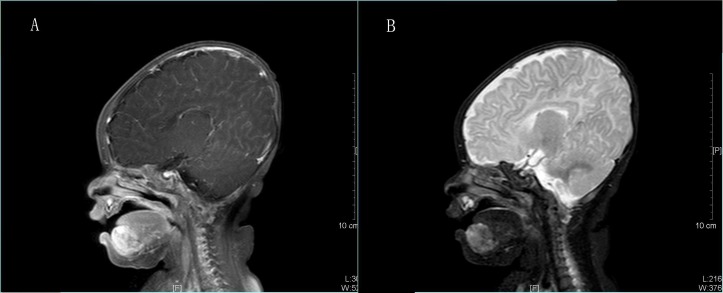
Magnetic resonance images of mandibular melanotic neuroectodermal tumour of infancy. (A) T1-weighted and (B) T2-weighted images.
